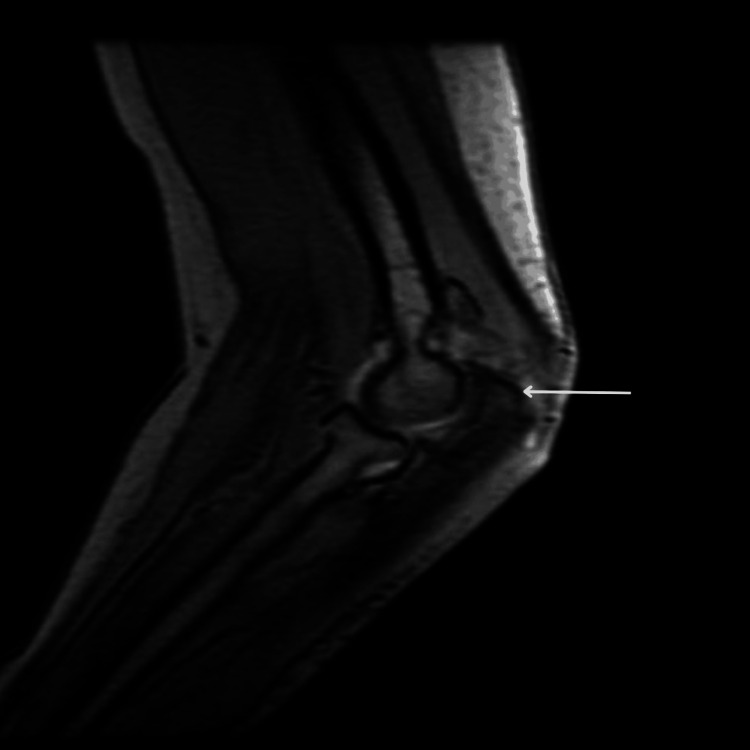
MRI showing heterotopic bone formations
